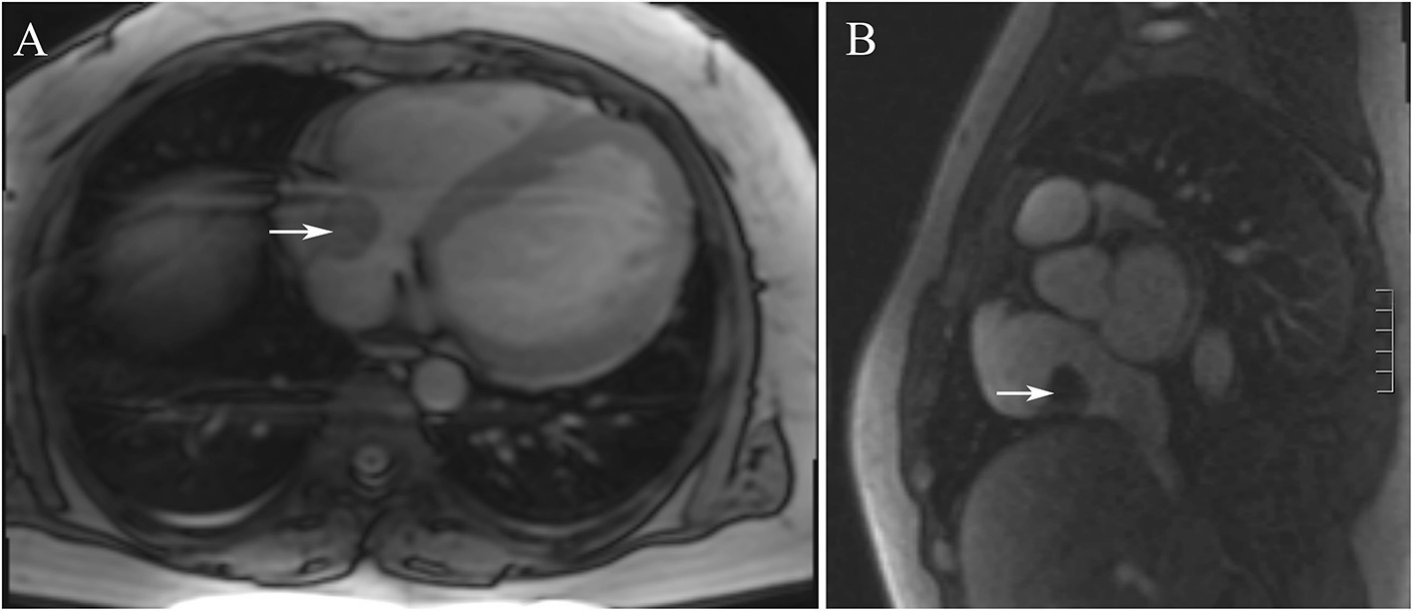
(A,B) The cardiac magnetic resonance imaging showed that showed that a well-circumscribed, homogenous “shadow” in the right atrium; it measured 29 mm × 27 mm, had equal T1- and T2-weighted signal intensities, was adjacent to the tricuspid valve.
